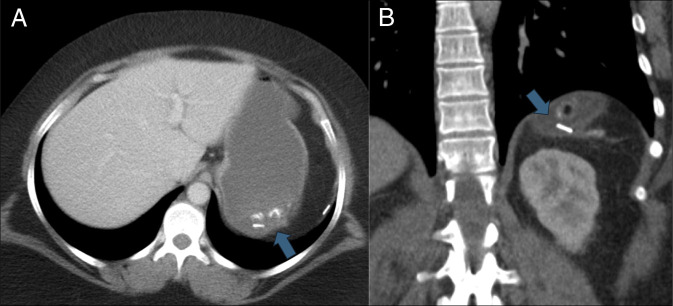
Axial (A) and coronal (B) computed tomography of the abdomen obtained with intravenous contrast and 900 mL of water by mouth show one of the splenectomy clips located inside the gastric lumen (blue arrow).
